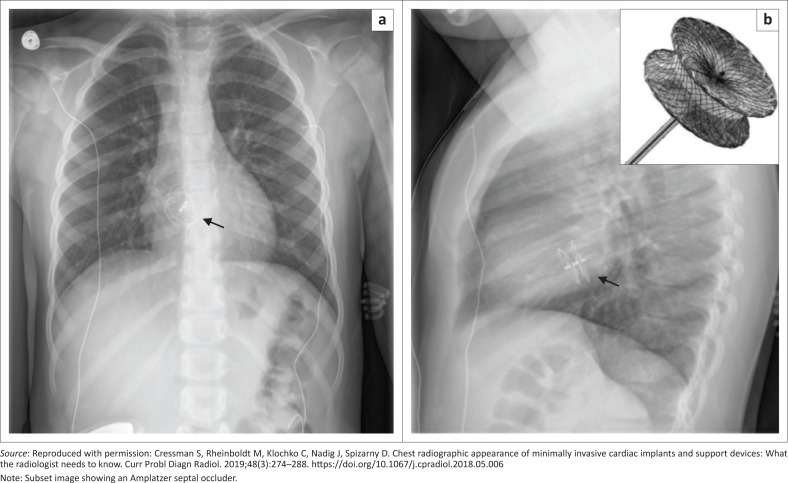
Chest radiographs showing a normally positioned Amplatzer septal occluder (arrow) in a 4-year-old girl with a history of a secundum atrial septal defect. Note that the ASO is projecting over the right lateral margins of the T8 vertebral body on the frontal view (a) and located anterior to the hilar-caval line on the lateral view (b).^16^
